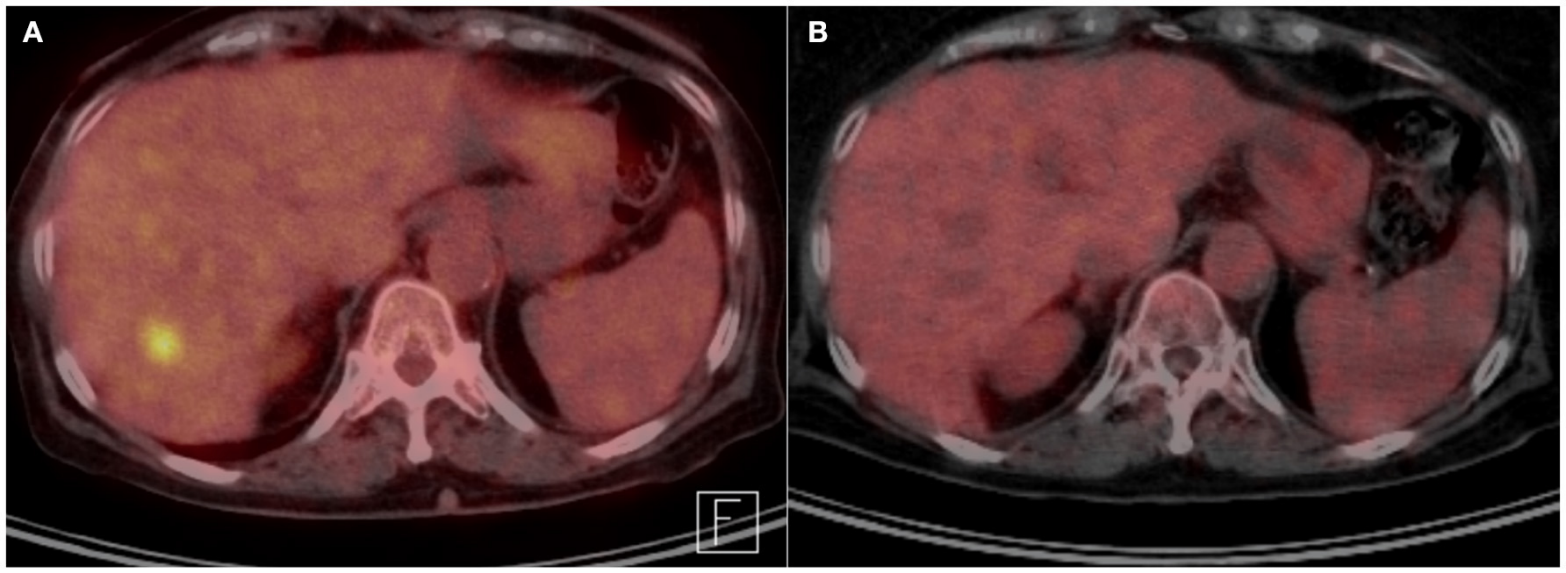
(A) Transverse abdominal PET scan before systemic therapy with TDM1, showing FDG avid liver lesion. (B) Transverse abdominal PET scan after treatment, showing disappearance of FDG avid liver lesion.
